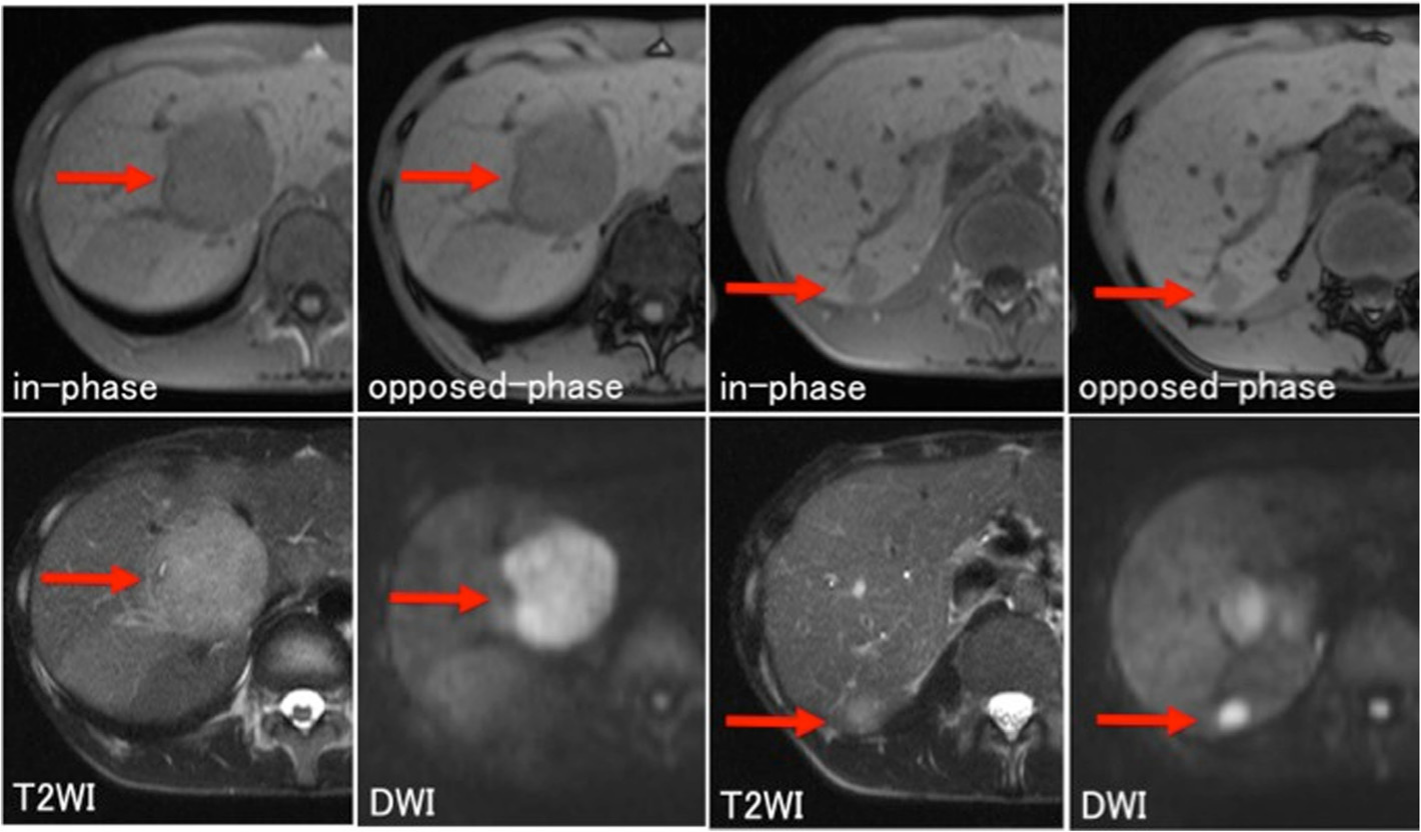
Abdominal magnetic resonance (MR) imaging. Abdominal magnetic resonance (MR) imaging revealed that the hepatic tumors (segment 5: arrow, segment 6: arrowhead) were slightly hypointense on T1-weighted imaging (WI), slightly hyperintense on T2WI, and hyperintense without an apparent fat component on diffusion-weighted imaging.
